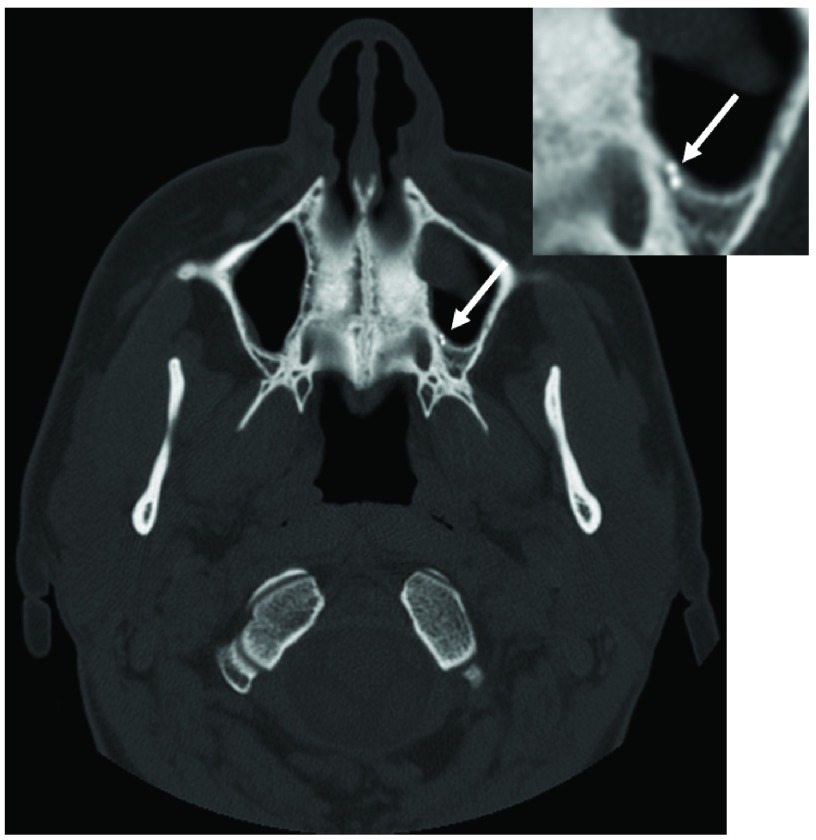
Axial section of non-contrasted CT scan shows two retained remnants of gutta-percha (white arrow) in the left posterior maxillary sinus. Inset in the top-right corner reveals a zoomed image of the two gutta-percha remnants.
